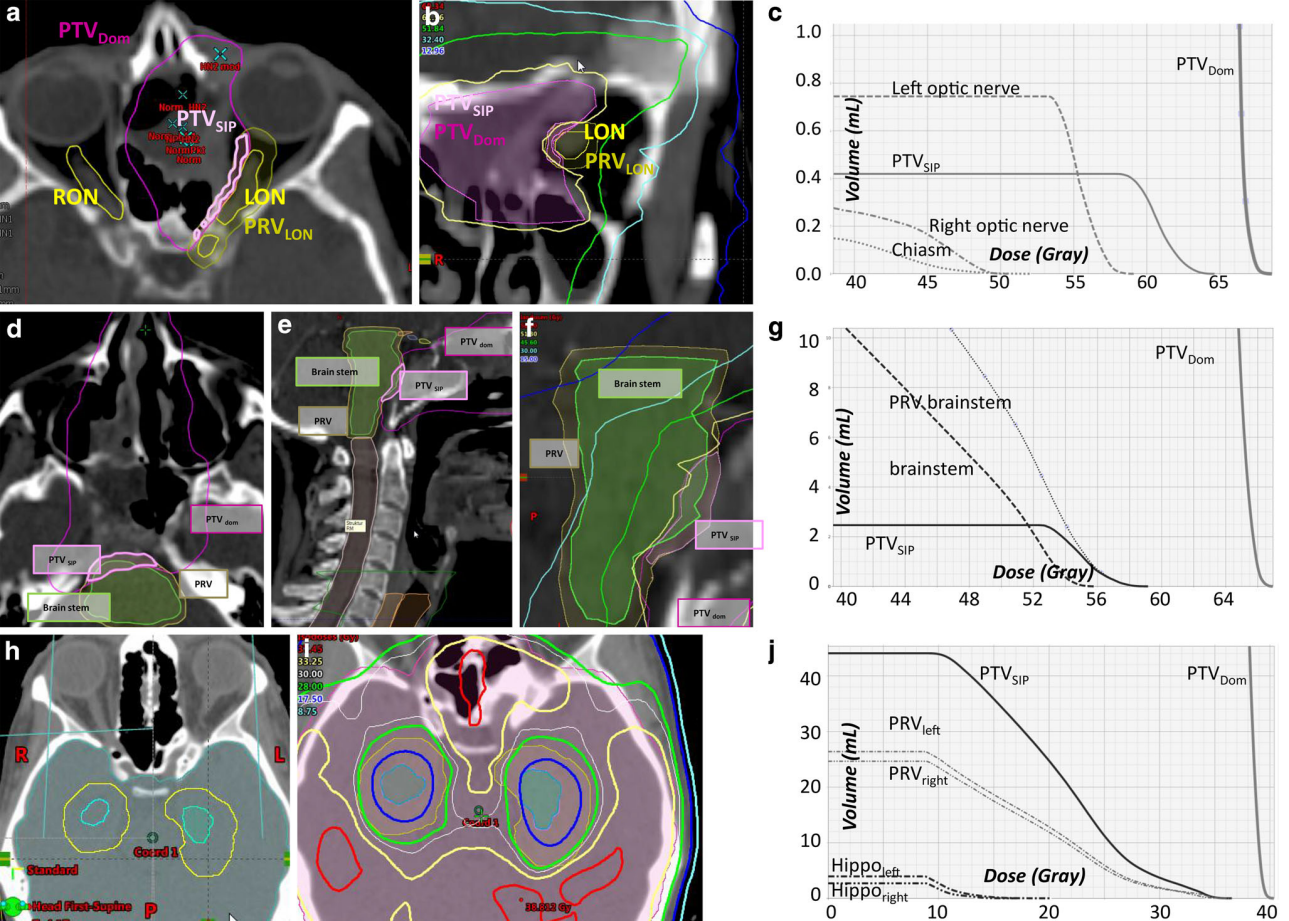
Examples for a simultaneous integrated protection (*SIP*) for the optic nerve, the brainstem, and the brachial plexus with dose parameters in Supplementary Table 2. a Axial planning CT of a patient with sinonasal squamous cell carcinoma who was treated with chemoradiotherapy after positive margin resection at the left optic nerve. As she refused left orbital exenteration, PTV_dom_ is treated with 64.8 Gy in 36 fractions. A 0.4 ml SIP volume is employed to respect a 60 Gy constraint to the left optic nerve. b The coronal plane visualizes the *yellow* 61.5 Gy isodose line around the nerve. c Absolute dose–volume histogram (*DVH*). d Axial and e sagittal planning CT of a patient with undifferentiated main nasal cavity carcinoma with initially direct contact to the brainstem which was shifted dorsally. Tumor shrinkage after two courses of induction chemotherapy with paclitaxel/cisplatin, then chemoradiotherapy with SIP-IMRT to 54 Gy during phase 1 followed by an adaptive sequential boost (not shown) during phase 2. f Isodoses at the interface between the PTV and the brainstem. g The brainstem constraint of 53 Gy is met as shown in the absolute DVH. h–j Hippocampus protection. h Delineation of the right (*sky blue*) and left (*blue-green*) hippocampus with the respective PRVs (*yellow*) that are generated by a 7 mm isotropic margin to the hippocampi. PTV_SIP_ corresponds to the PRV of the hippocampus minus the hippocampus itself (PTV_SIP_ = PRV_[side]_\hippocampus_[side]_). i A total dose of 35 Gy in 14 fractions was prescribed to the PTV_dom_. Note the 28.0 Gy (*green*) and the 17.5 Gy (*cornflower blue*) isodoses at the two SIP volumes. j In the absolute DVH, the hippocampi receive a mean dose of ≤10 Gy. *PRV* planning risk volume, *PTV* planning target volume
